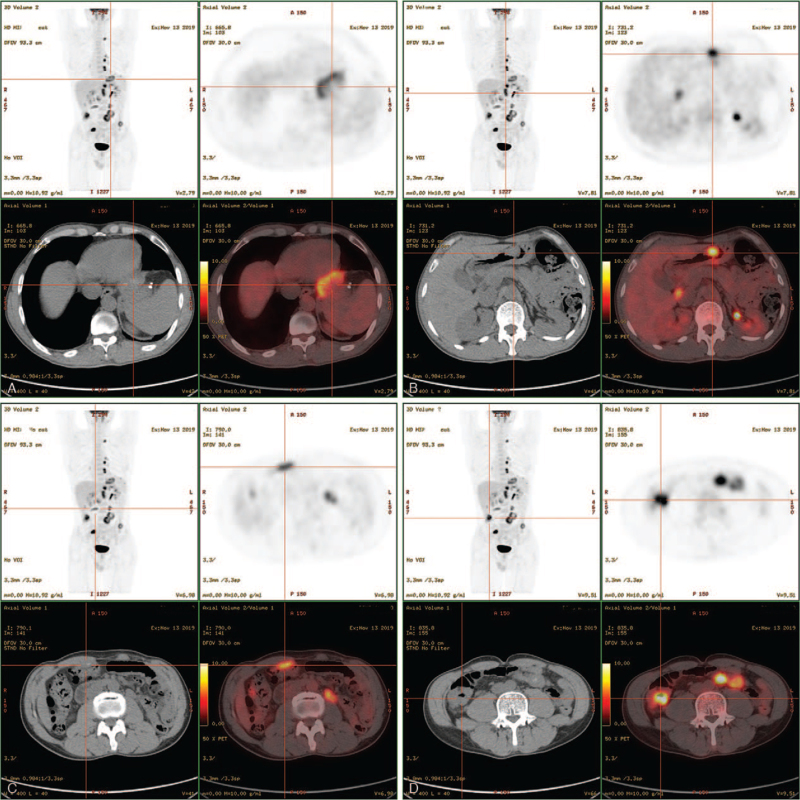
Increased FDG uptake under the diaphragm (A), in the corpus of the stomach (B), in the mesentery (C), and in the thickened parts of ileum and sigmoid (D).
